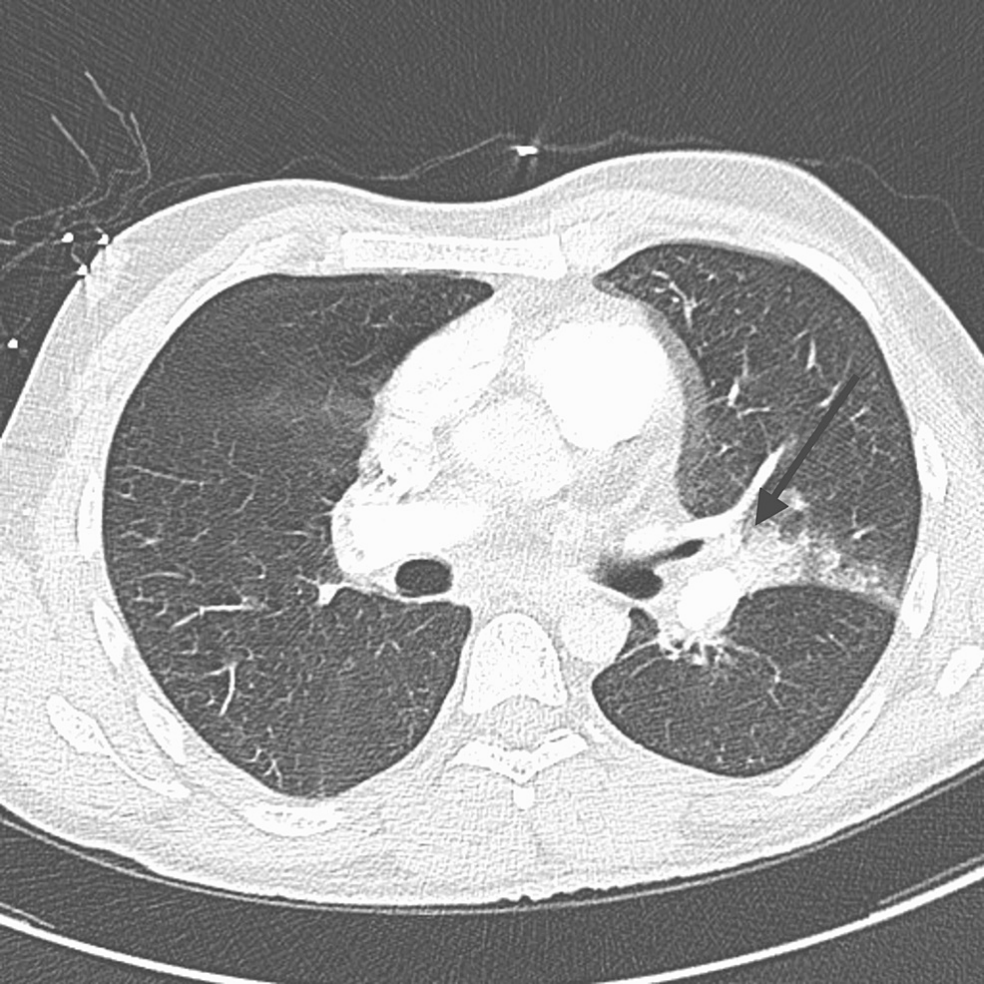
High-resolution computed tomography (HRCT) scan showed redemonstration of an extensive PE with right ventricular strain PE: pulmonary embolism
